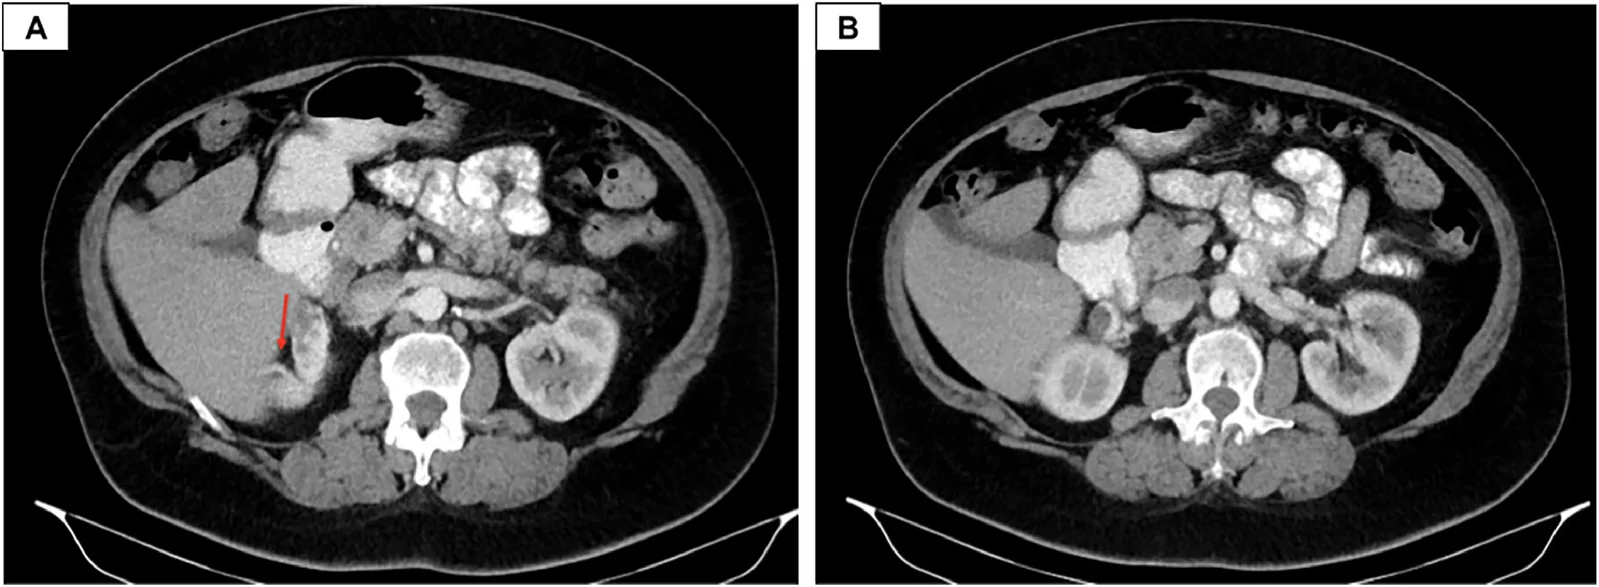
(A) Axial portal venous-phase CT demonstrates the right perirenal lesion; the arrow highlights an associated enhancing renal capsular vein. (B) Follow-up axial CT obtained 9 months after the initial CT demonstrates persistence of the mass adjacent to the right kidney without interval growth.
